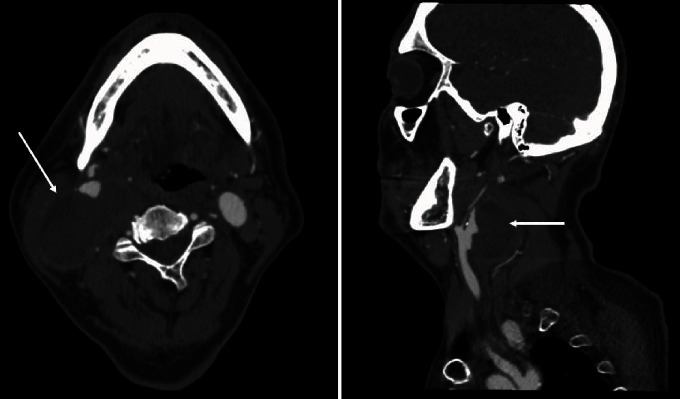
Computed Tomography (CT) scan shows a 60 × 10^5^ mm pseudoaneurysm of the right carotid bifurcation (arrows).
